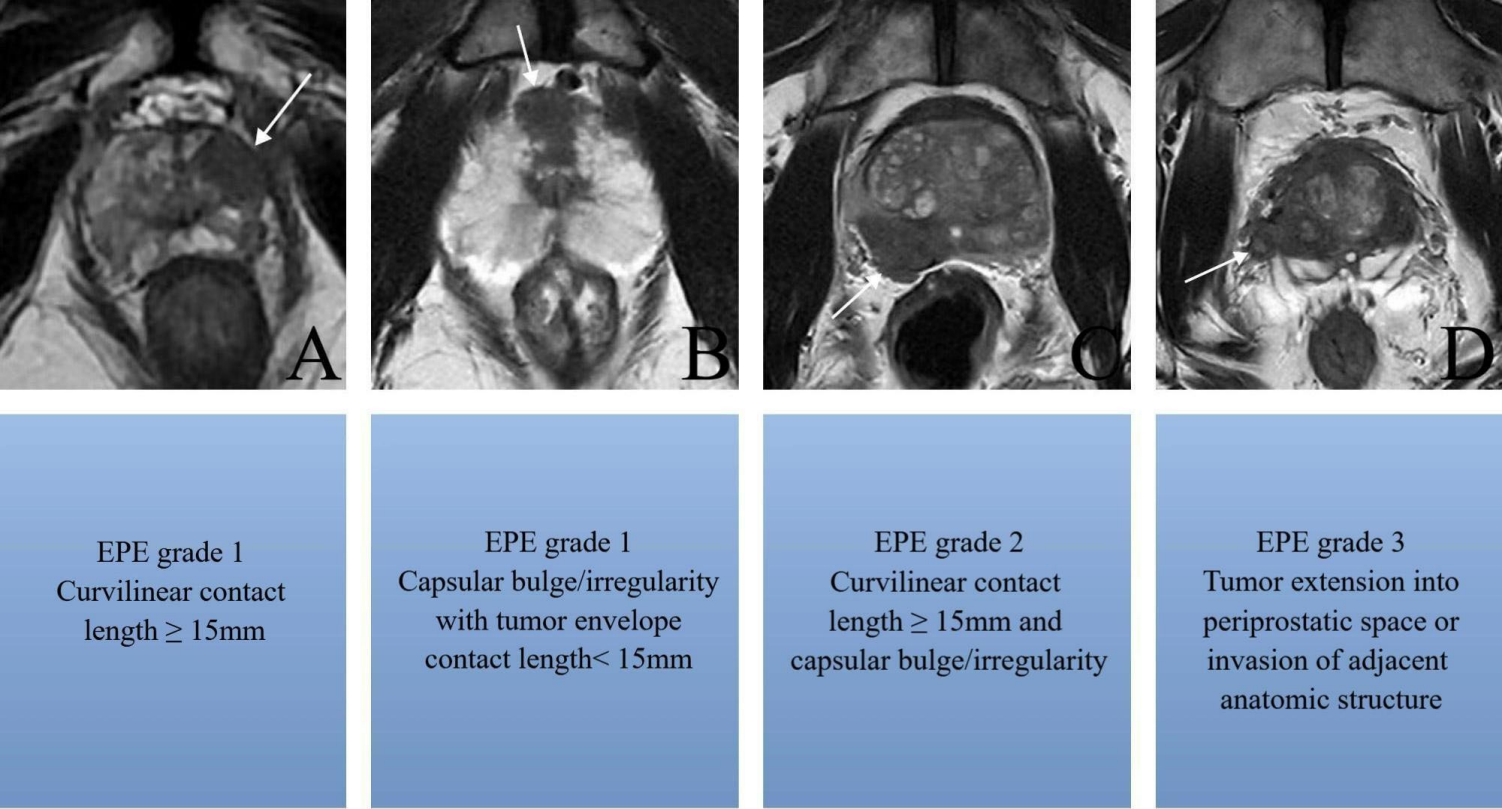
Axial T2-weighted MR images show (A) tumor envelope contact length ≥ 15mm without irregular envelope (arrow), (B) envelope swelling and irregular envelope with tumor envelope contact length< 15mm (arrow), (C) ≥15 mm contact length of the envelope with irregular and bulging envelope(arrow), (D) tumor extension into periprostatic space or invasion of adjacent anatomic structure in magnetic resonance images (arrow).
